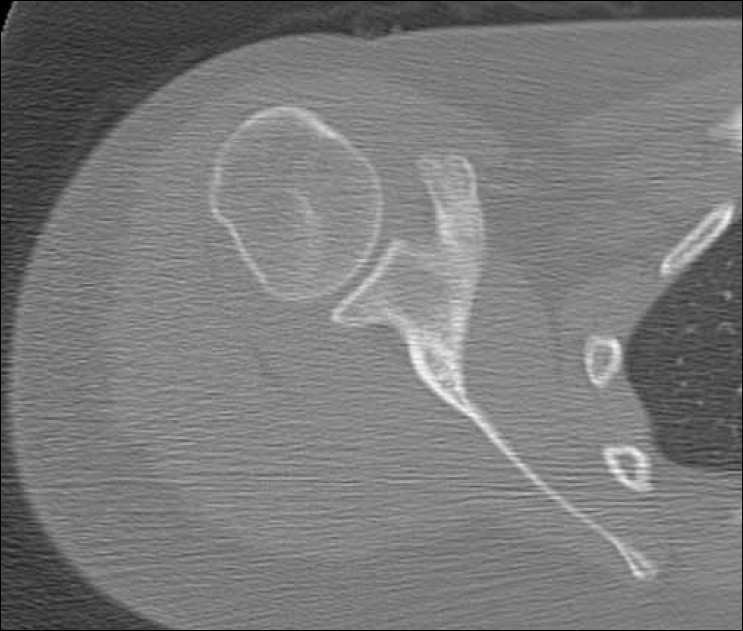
Axial computed tomography image demonstrates healing of the fracture at 3 months postinjury
